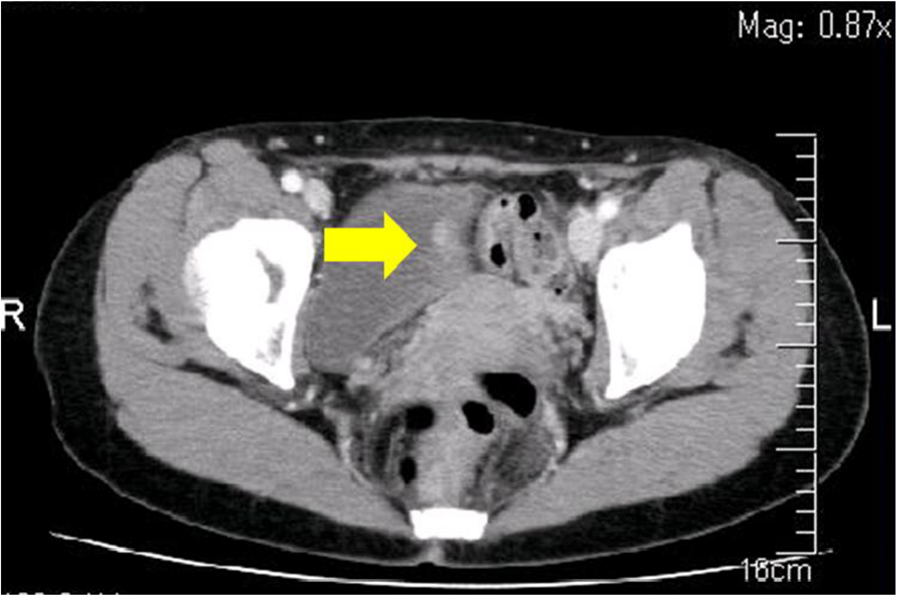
Abdominal computed tomography showing a 6mm mass in the bladder.
